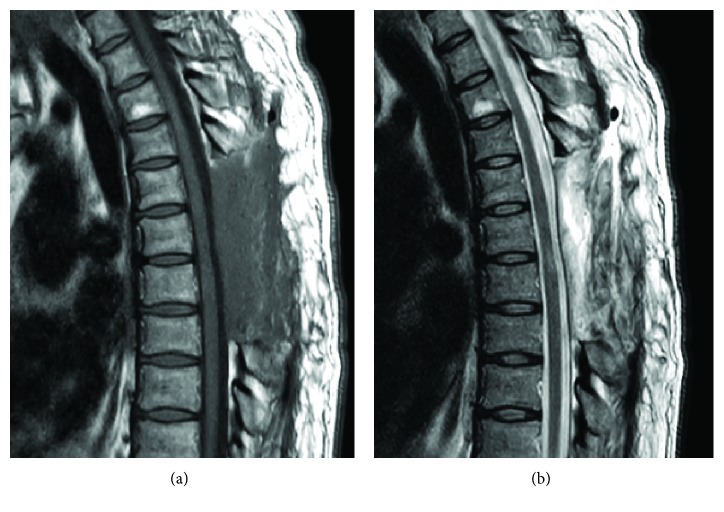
Postoperative thoracic MRI (seven days after surgery). Postoperative MRI showed the complete resection of the tumors. Sagittal view of the T1-weighted image (a) and T2-weighted image (b).
